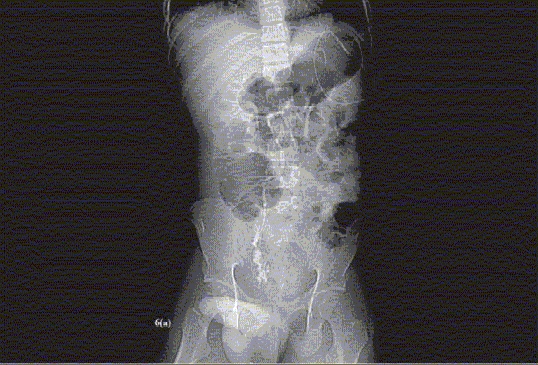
KUB, CT scan, Retrograde Pyelogram, Antegrade Nephrostogram and Rotational Fluoroscopic Tomography. The patient is a teenage male who had sustained penetrating trauma to his perineum. A tree branch penetrated through his perineum, into the right lower quadrant of the abdomen, avulsing his right ureter from the bladder at the UVJ. (a) KUB obtained after IV contrast administered for CT scan. Image demonstrates extravasation of contrast from the distal right ureter
